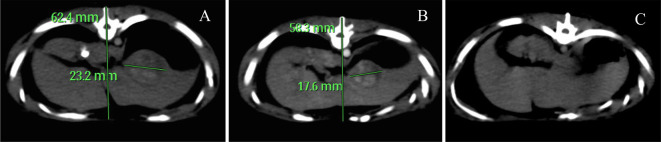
Tracking the response to proton beam treatment with multiphase contrast-enhanced CT: (A) At the end of treatment, (B) 3 weeks after treatment, (C) 9 weeks after treatment.
